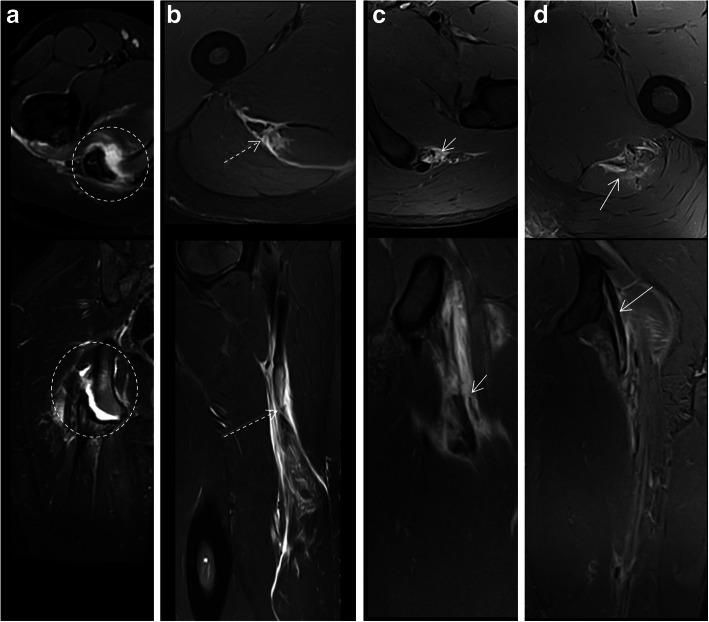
Axial and coronal/sagittal MR images in fluid-sensitive sequences of the proximal hamstring complex tendon. a Complete apophyseal avulsion fracture of the right ischial tuberosity with severe increase of the signal intensity at the avulsion site (dashed circles). b Complete transverse tear (dashed arrows) of the common tendon of the biceps femoris and the semitendinosus with thickening and distal retraction. c Complete avulsion of the semimembranosus proximal tendon (short arrows) with retraction. d Mixed partial rupture of the semimembranosus proximal tendon
